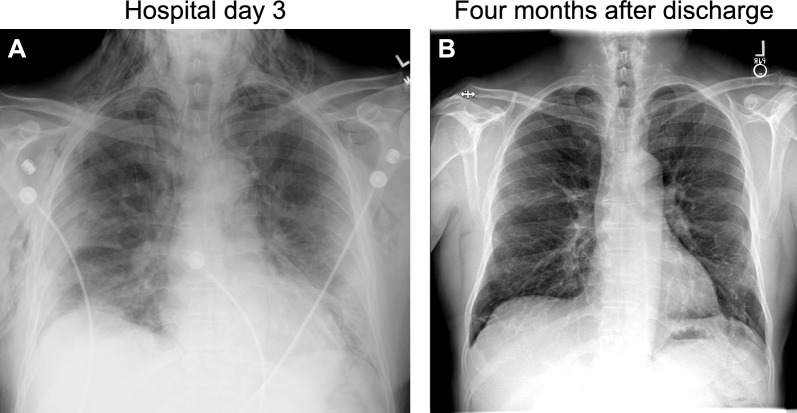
Chest radiography on hospital day 3 (A) and at 4 months after discharge (B). (A) The radiography was obtained on hospital day 3 after the patient developed a “popping sensation” in the neck, showing diffuse bilateral lung infiltrates and extensive subcutaneous emphysema. There was widening of the mediastinum consistent with pneumomediastinum, although it could have been positional. (B) At 4-month follow up, the radiography showed significant improvement of bilateral lung infiltrates and complete resolution of subcutaneous edema
